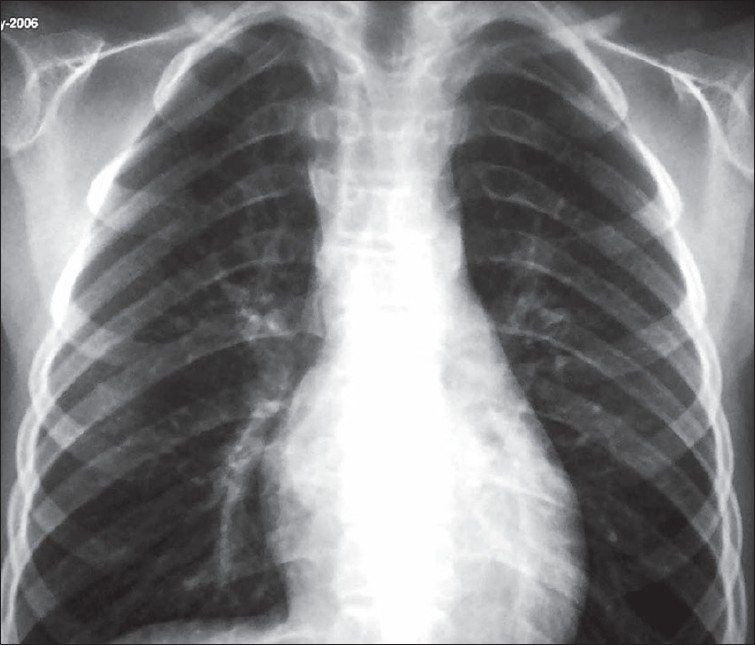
Radiograph of the chest shows absence of the lateral half of the clavicle and thin ribbon-like third rib on both sides
